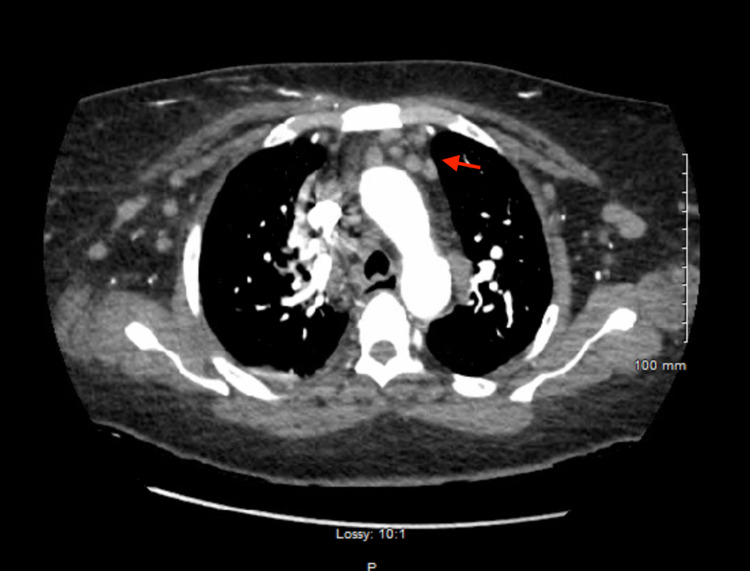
CT pulmonary angiography with contrast showing hilar and mediastinal lymphadenopathy (red arrow).
